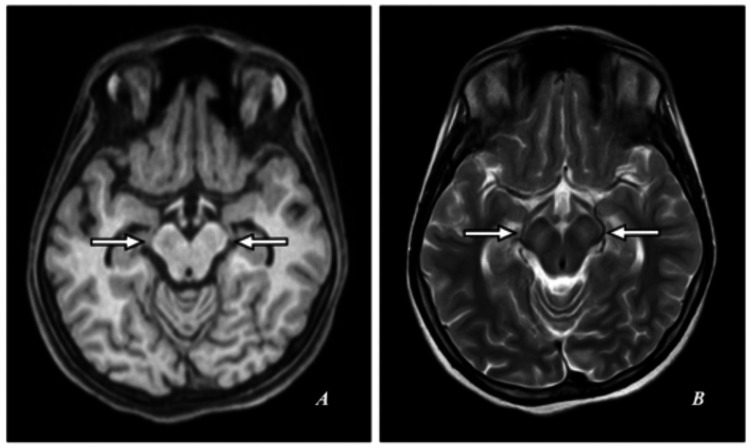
MRI of the brain. (A) T1-weighted (T1W) axial image. (B) T2-weighted (T2W) axial image. T1W: Symmetrical subtle hypointensities. T2W: Hyperintensities in the midbrain tegmentum with preservation of normal signal intensities of the lateral aspect of pars reticulata of substantia nigra, resembling the appearance of “ears,” normal signal intensities of the red nucleus, resembling the appearance of “eyes,” and hypointensity in the superior colliculus resembling the appearance of “chin,” showing the “face of a giant panda sign” (short white arrows).
